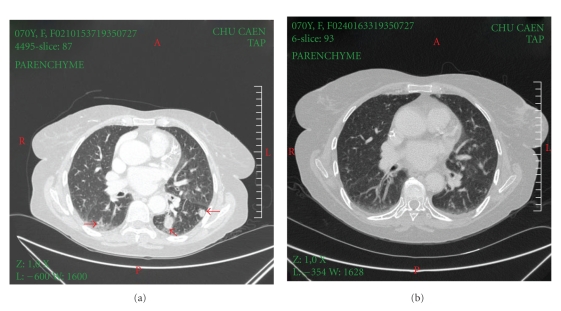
Pulmonary localisation of lymphomatoid granulomatosis. Transverse CT view, with contrast injection in parenchymatous window showing nodular lesions taking contrast. (a) At initial diagnosis. (b) 2 years after reduction in the immunosuppression and rutiximab therapy.
